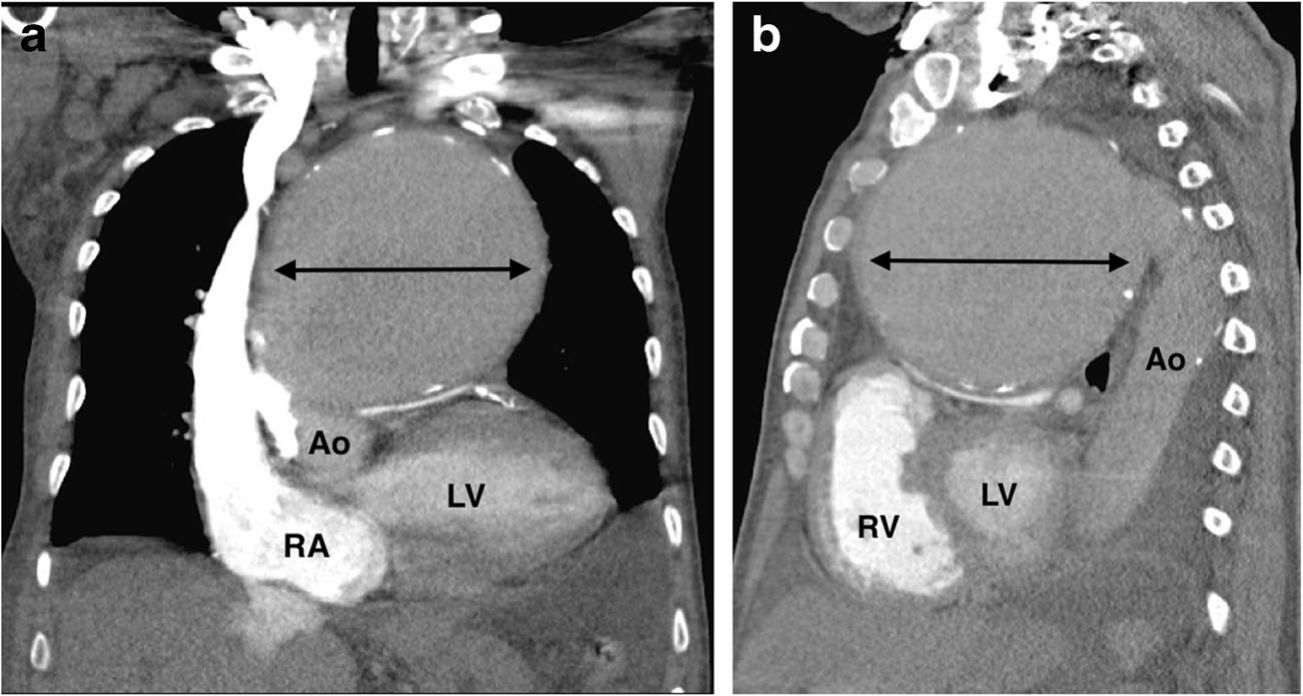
A 70-year-old-man with chest pain and dyspnoea on exertion underwent a CT pulmonary angiogram (CTPA) for suspected pulmonary embolus. (a) Coronal and (b) sagittal MPRs from the CTPA demonstrate a large, fusiform, peripherally calcified aneurysm (*double arrow*) of the ascending aorta (Ao), causing severe compression of the main pulmonary artery inferiorly and abutting the sternum anteriorly. The patient underwent surgical repair, with pathology-demonstrated classical appearance of syphilitic aortitis, caused by the spirochete *Treponema pallidum. RA = right atrium; RV = right ventricle; LV = left ventricle*
